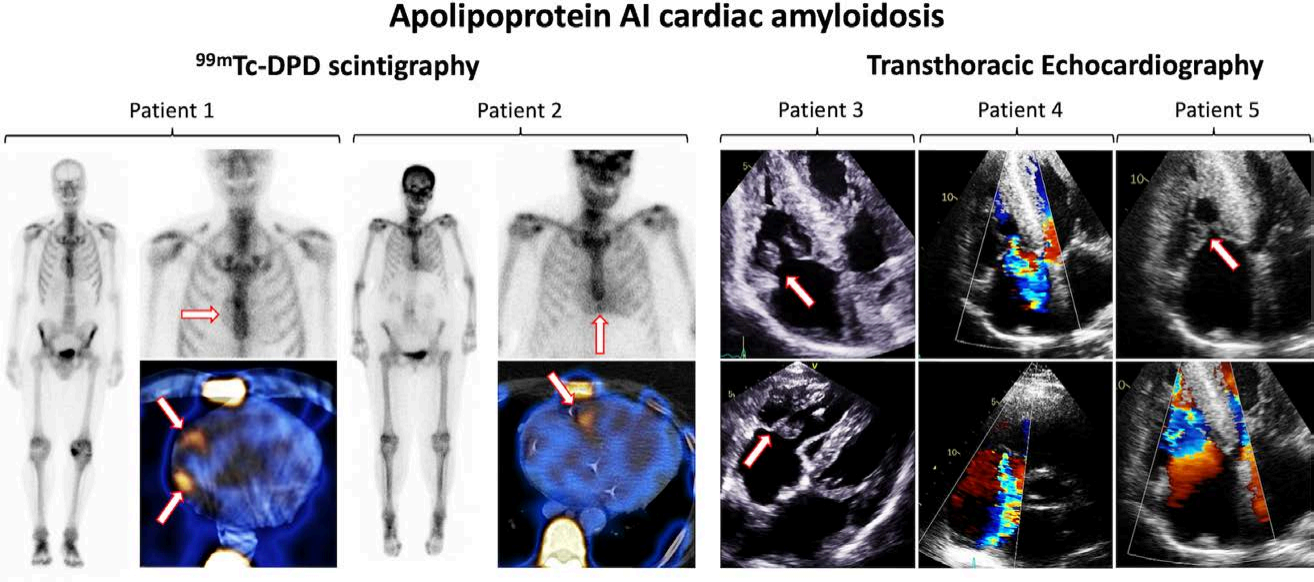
^99m^Technetium labeled 3,3-diphosphono-1,2-propanodicarboxylic acid (^99m^Tc-DPD) scintigraphy and echocardiographic images of patients with Apo AI cardiac amyloidosis. Patient 1=^99m^Tc-DPD scintigraphy and single-photon emission computed tomography (SPECT) images demonstrating grade 1 cardiac uptake with prominent right atrial uptake (arrows). Patient 2=^99m^Tc-DPD scintigraphy and SPECT images demonstrating grade 1 cardiac uptake with prominent right ventricular uptake (arrows). Patient 3=Transthoracic echocardiography demonstrating marked thickening of the tricuspid valve leaflets (arrows) and subvalvular apparatus and biventricular hypertrophy. Patient 4=Transthoracic echocardiography demonstrating severe tricuspid regurgitation. Patient 5=Transthoracic echocardiography demonstrating tricuspid stenosis (arrows), with turbulent forward flow through the tricuspid valve, biatrial enlargement, and biventricular hypertrophy.
